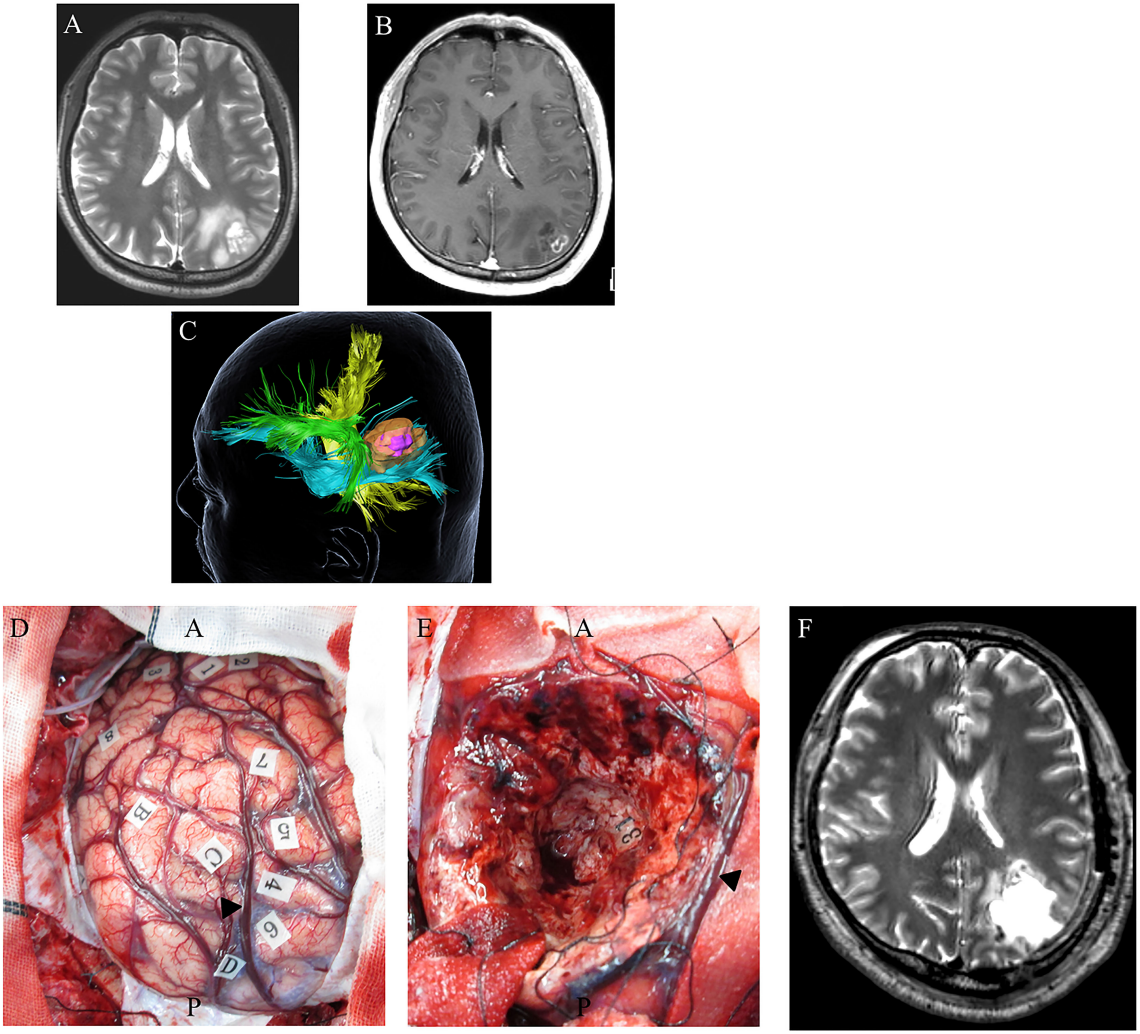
A 48-year-old right-handed male with *IDH*-wildtype glioblastoma (GBM). Preoperative axial T2-weighted (A) and axial T1-weighted MRI with gadolinium enhancement (B), showing a high-intensity abnormal area in the left inferior parietal lobule including an enhancing region at the superficial area. Preoperative three-dimensional tractography (C) showing a yellow-colored fiber tract bundle showing the corticospinal tract. The green area shows the superior longitudinal fasciculus, the blue fiber tract bundle shows the inferior fronto-occipital fasciculus, and the high-intensity area on T2-weighted MRI is highlighted in orange. The violet-colored lesion in the orange-colored area showed an enhancing mass. (D) Intraoperative photograph obtained before tumor resection, with letter tags indicating tumor boundaries (A–D). Stimulation over the postcentral gyrus induced convulsions around the mouth (number tags: 1, 2); stimulation over the left superior parietal lobule induced cessation of right upper limb movement (number tags: 4–7); arrowhead: intraparietal sulcus. (E) Intraoperative photograph obtained after supratotal resection. Stimulation over the IFOF induced semantic paraphasia (number tag: 31). Postoperative axial T2-weighted MRI (F) revealed no tumor following supratotal resection.
